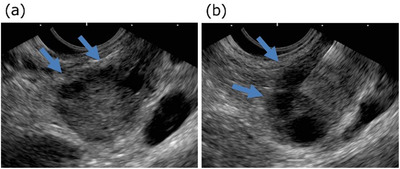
(a) Endoscopic ultrasound showed a 20‐mm‐diameter low‐echoic tumor in the pancreatic tail. There were multiple cystic lesions at the tumor margins, which were thought to be dilated branched pancreatic ducts (arrows). (b) A transgastric puncture was performed with a 22‐G Franseen needle (Acquire, Boston Scientific). The puncture site was close to the dilated branched pancreatic ducts (arrows)
